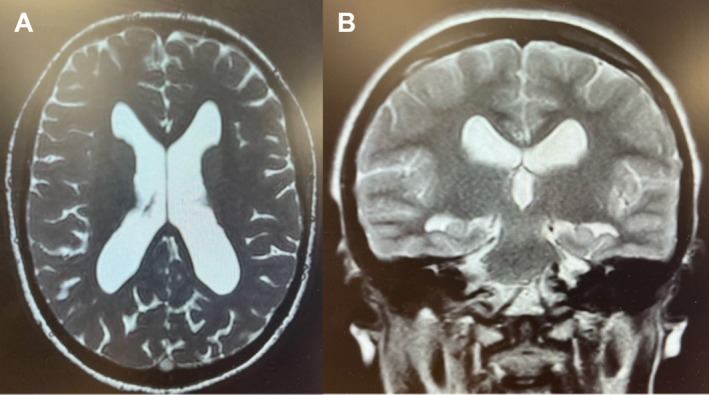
Postoperative axial (A) and coronal (B) MRI slices demonstrating reduced ventricular size following endoscopic third ventriculostomy.
